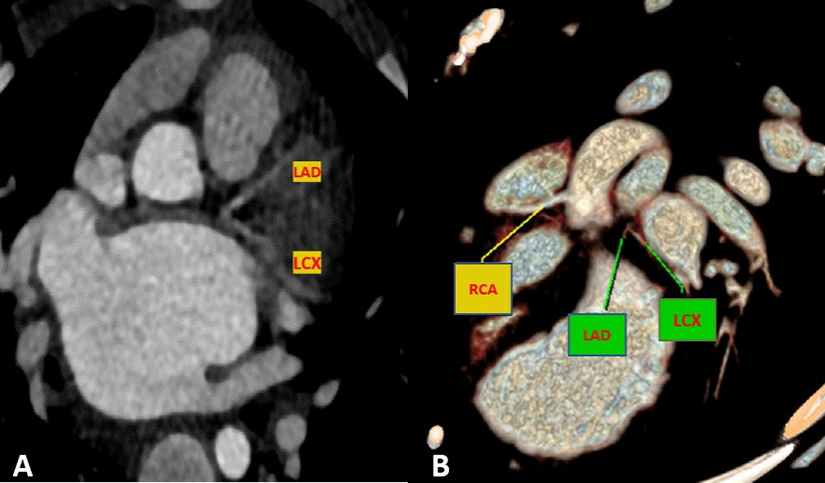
Maximum intensity projection (A) and volume rendered (B) CT images demonstrate an absence of the LM artery with proximally connected left anterior descending artery (LAD) and left circumflex artery (LCX).
